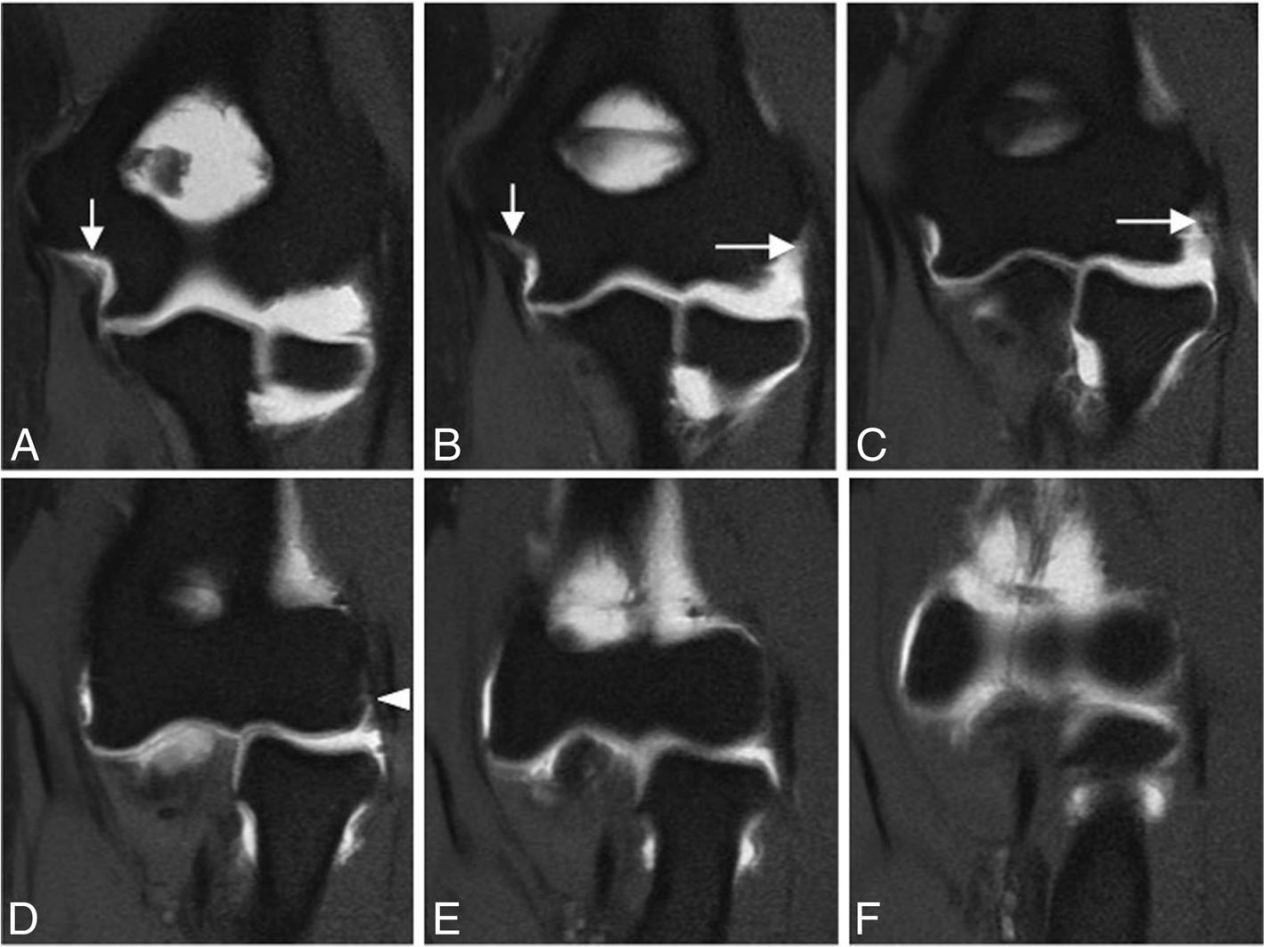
Posterolateral rotatory instability, stage 3B. Consecutive coronal FS T1-weighted direct MR arthrographic images (a–f) showing a proximal partial-thickness tear of the anterior bundle of the medial collateral ligament complex (short arrows), proximal complete tear of the lateral ulnar collateral (long white arrows), and partial-thickness tear of the radial collateral ligament proper (white arrowhead)
